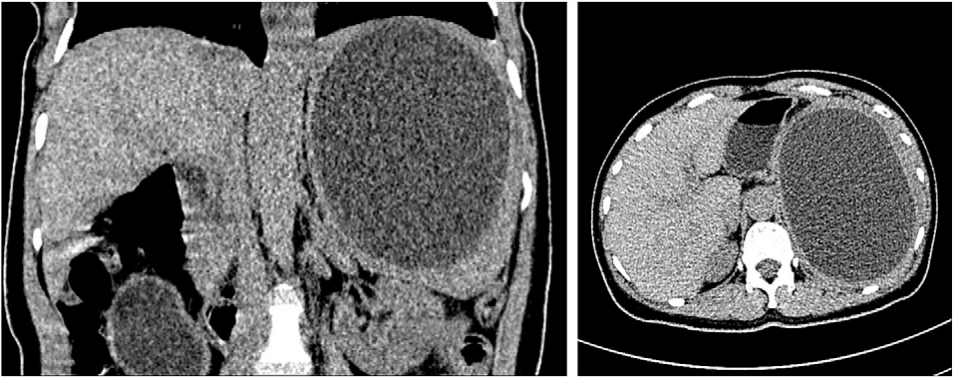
The coronal view (on left) and axial view (on right) of computed tomography showing a 15.2 × 14-cm–sized well-defined cystic lesion in the upper pole of the spleen.
